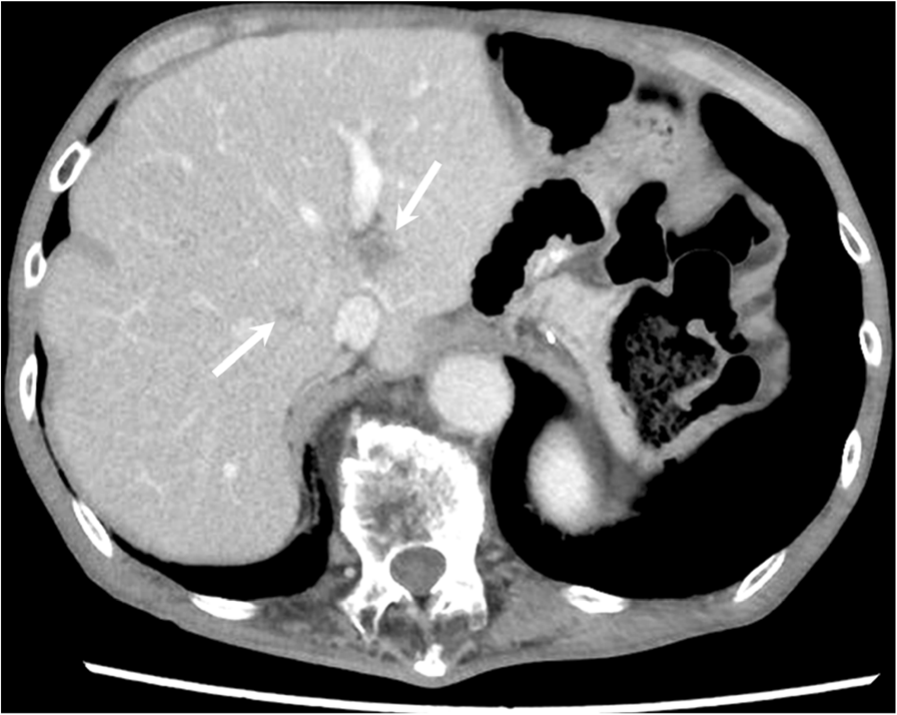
Abdominal computed tomography (CT) findings after four cycles of nivolumab. Abdominal enhanced-contrast CT revealed a marked reduction (by 55%) in liver metastases (arrows)
